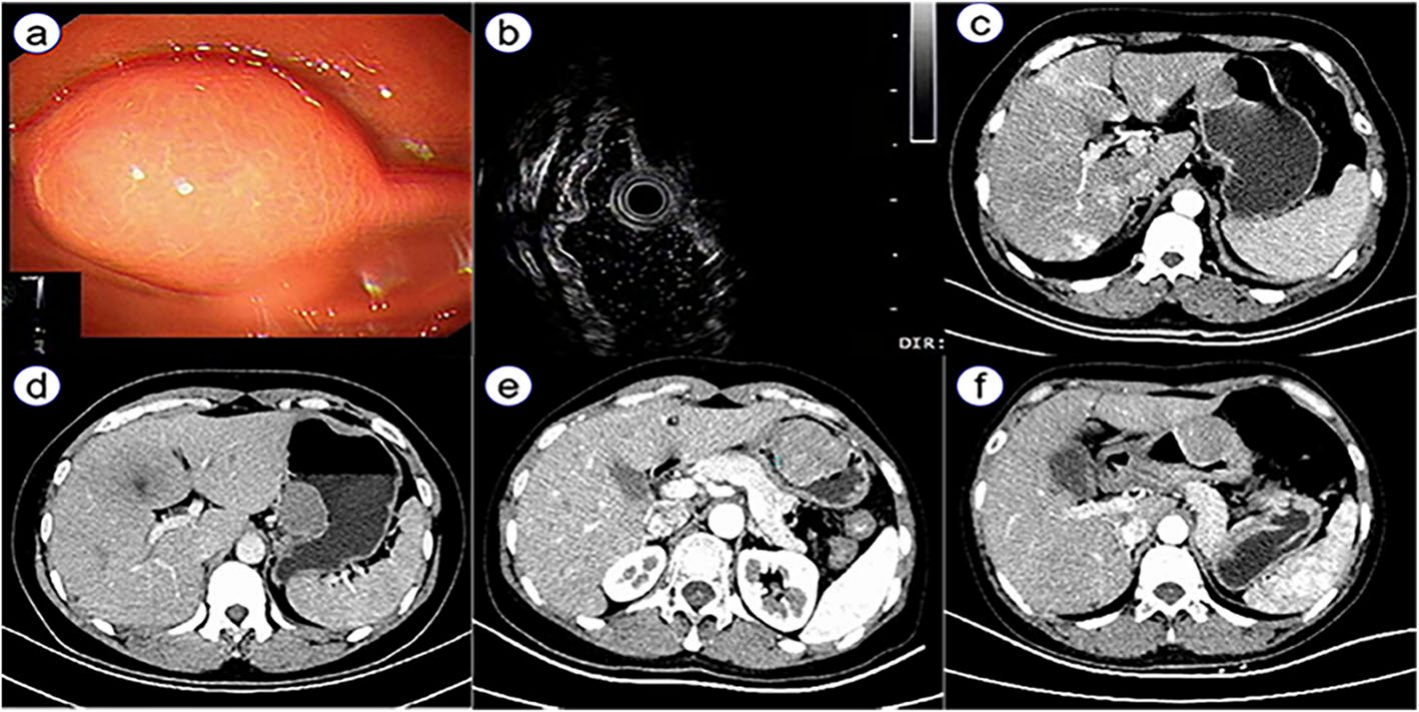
The diagnostic of characteristic imaging of gastric schwannomas. a endoscopy present as a smooth, round, elevated submucosal masses; b hypoechoic muscularis propria lesions with clear margin; c the mass with slight uniform enhancement CT; d another mass with slight uniform enhancement CT; e the mass with moderate uniform enhancement CT; f another mass with moderate uniform enhancement CT
